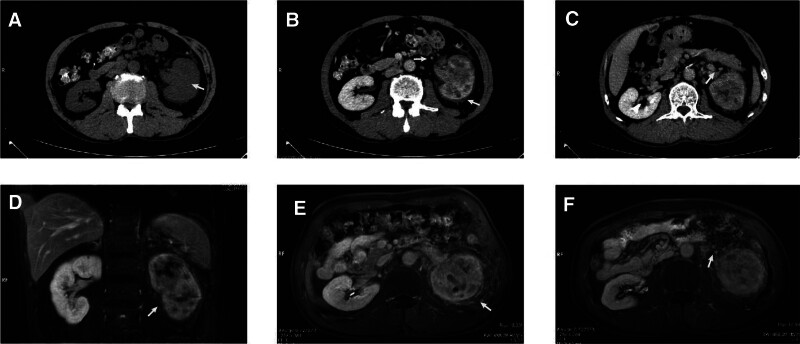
(A) CT showed increased volume of the left kidney, with no mass shadow seen. (B) CT showed thickening of the left perirenal fascia, blurring of the left perirenal fat, and multiple enlarged left retroperitoneal lymph nodes. (C) CT showed a suspected embolism in the left renal vein. (D, E) MRI showed an irregular shape of the left kidney, an uneven signal of the left kidney parenchyma, and a thickening of the perirenal fascia. (F) MRI showed multiple lymph node enlargement in the left retroperitoneum. The white arrows in the figure indicate the location of the lesion. CT = computed tomography, MRI = magnetic resonance imaging.
